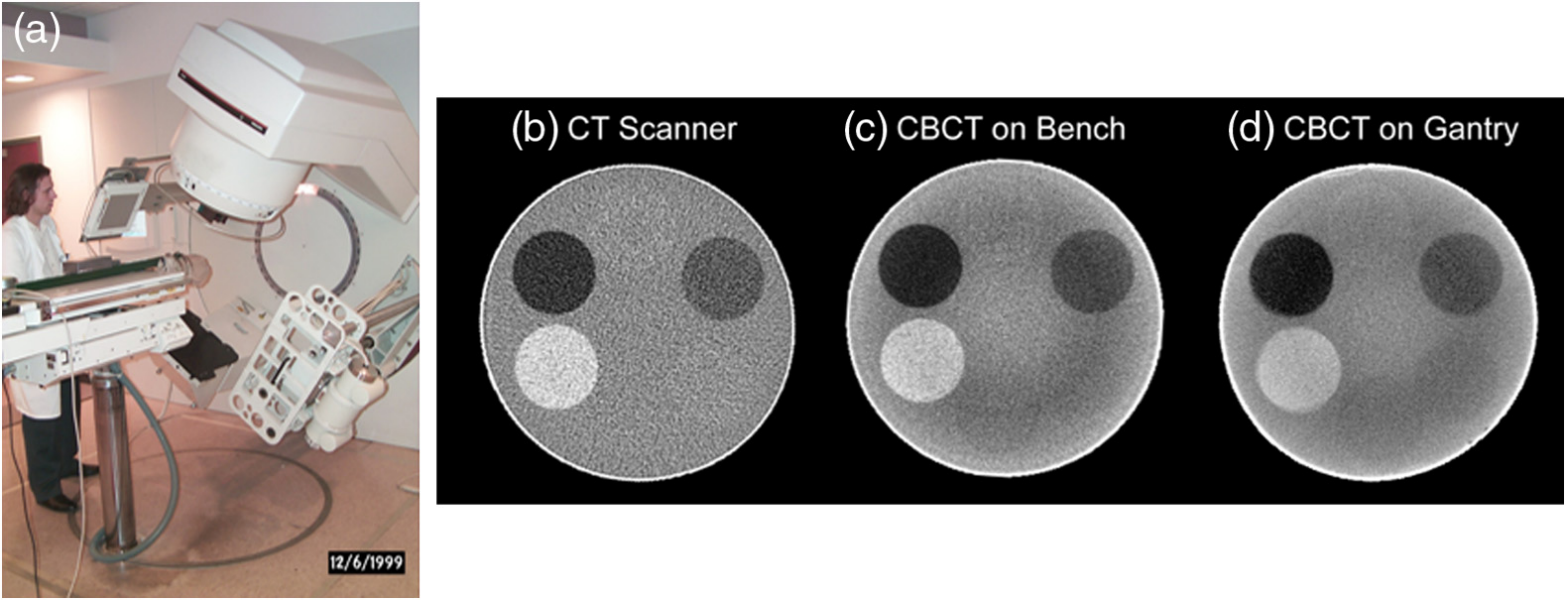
(a) Adaptation of the 20.5-cm FPI to the linear accelerator gantry for studies of image quality and geometric stability. (a)–(c) Comparison of soft-tissue performance between the clinical CT scanner (Philips SR-7000), bench-top, and gantry-mounted systems (1-mm effective slice thickness).
